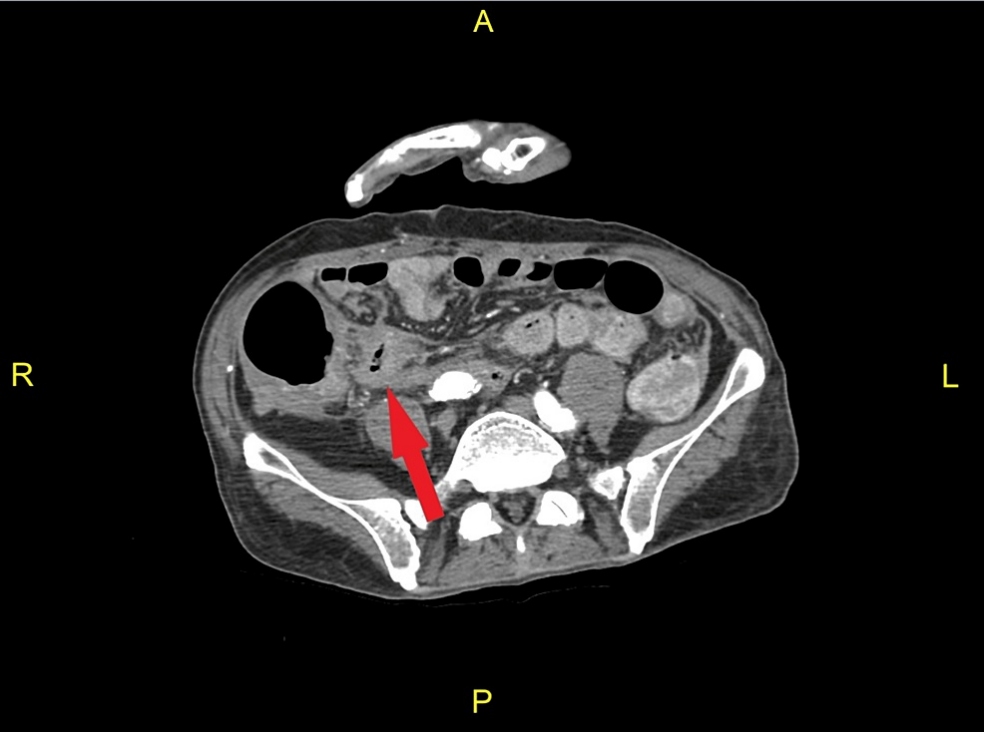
A CT angiogram of the abdomen and pelvis (axial section) Apparent long-segment mucosal thickening of the terminal ileum (red arrow) suggests chronic inflammation, possibly Crohn’s disease. There is no identified source of active bleeding. There is abundant fecal material throughout the rectum and sigmoid colon.
